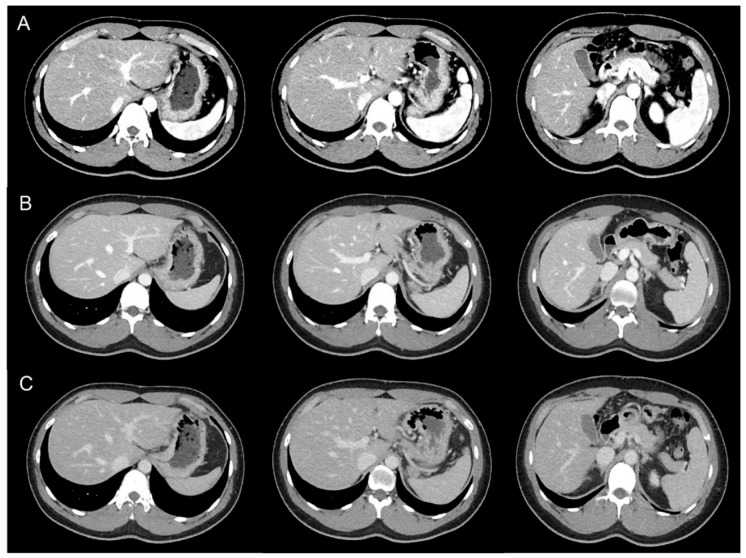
Contrast-enhanced dynamic liver CT showing diffuse, moderate hepatic steatosis without focal lesions. (A) Arterial phase, (B) portal venous phase, and (C) delayed phase.
